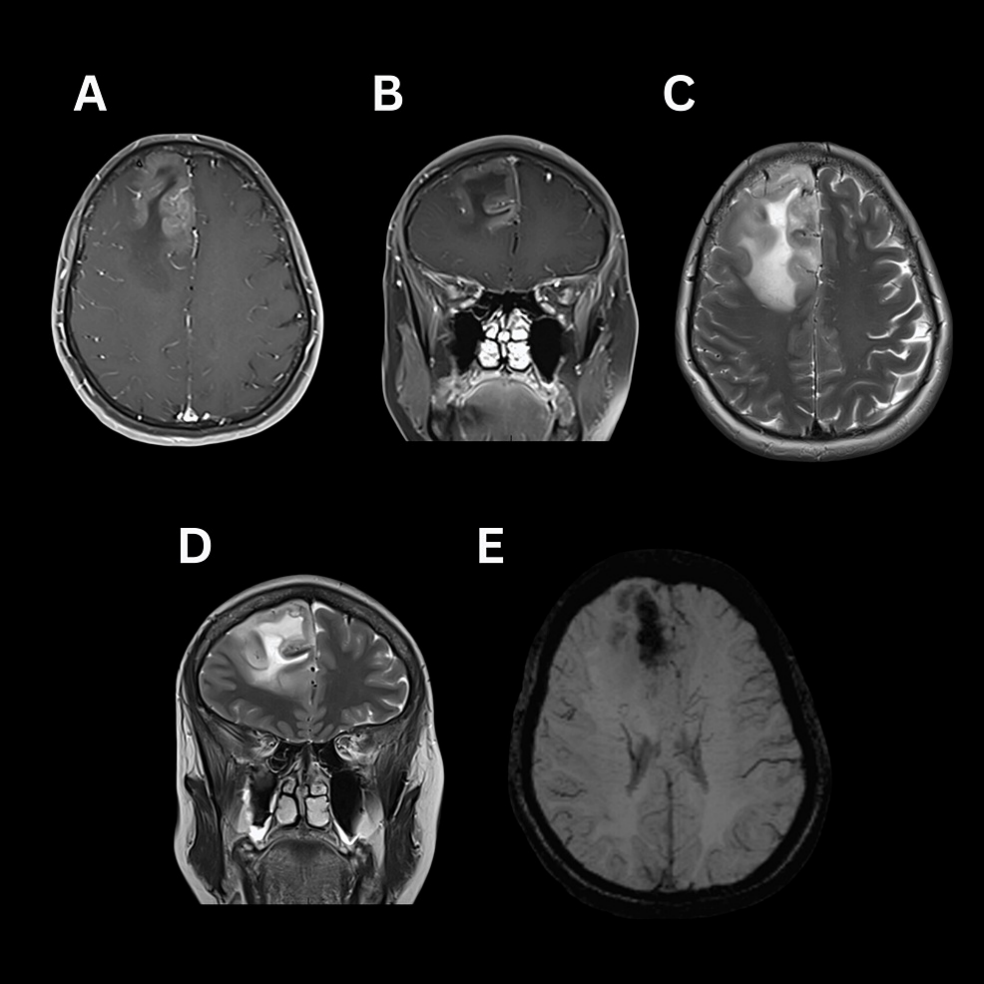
Brain MRI demonstrating hypointense lesion in T1 and hyperintense in T2, with blooming artifact in SWI (A: T1+Contrast Axial; B: T1+Contrast Coronal; C: T2 Axial, D: T2 Coronal; E: SWI). SWI: susceptibility weighted imaging
